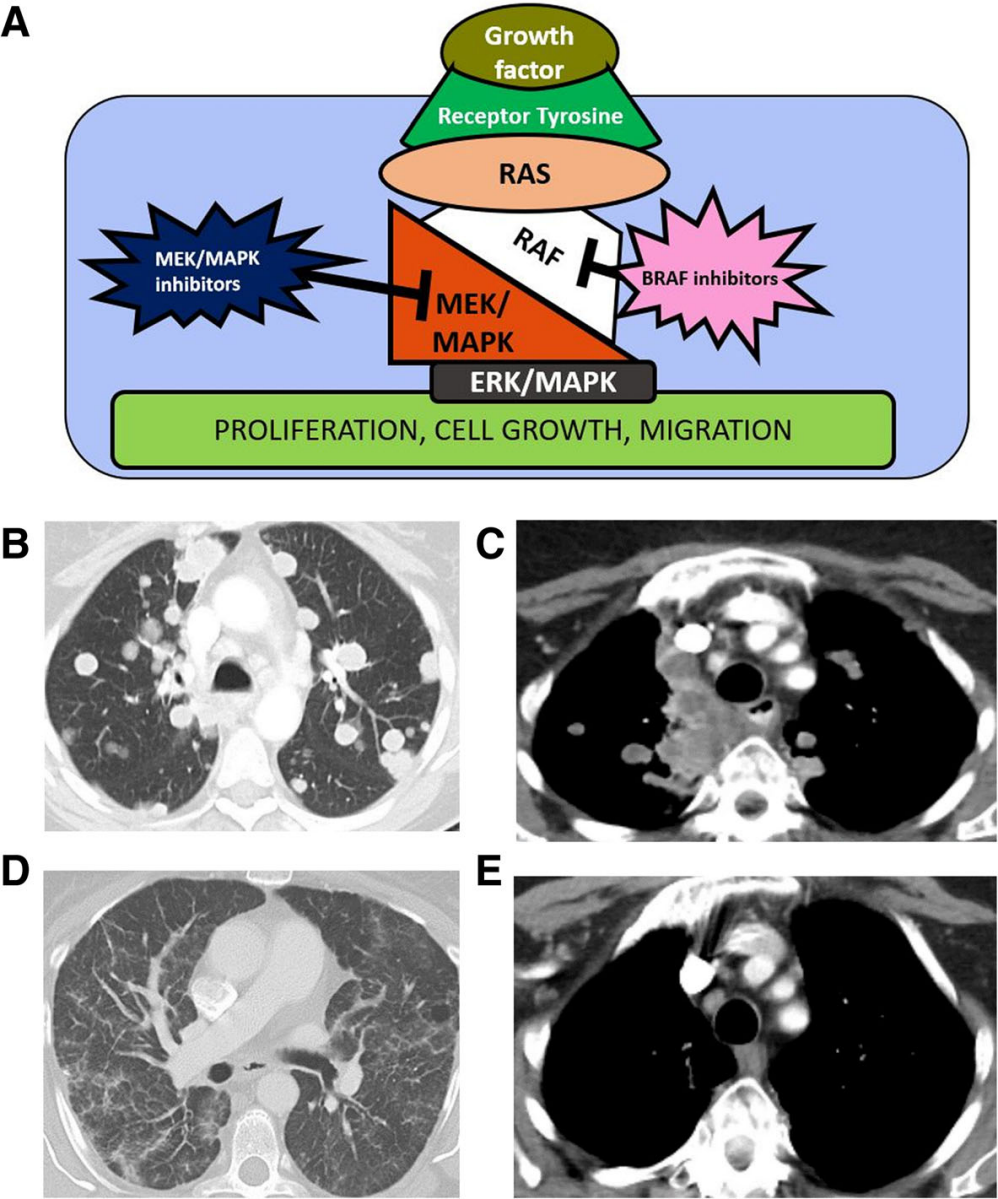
RTK-RAS pathway. a Image showing the activation of the MAPK transcription factor by binding of growth factor to a transmembrane receptor tyrosine kinase. The resulting signaling cascade culminates with translocation of ERK/MAPK to the nucleus, where ERK activates transcription factors that ultimately result in cell growth. BRAF inhibitors, such as dabrafenib, block RAF signal and MEK/MAPK inhibitors, such as trametinib, block the MEK/MAPK signal. a–d Contrast-enhanced CT images of the chest of a 52-year-old woman with BRAF V600 mutant melanoma, progressed on ipilimumab, treated with dabrafenib and trametinib. Baseline (a, b) and follow-up CT (c, d) performed two months after treatment was started, shows almost complete resolution of multiple lung nodules and mediastinal masses
